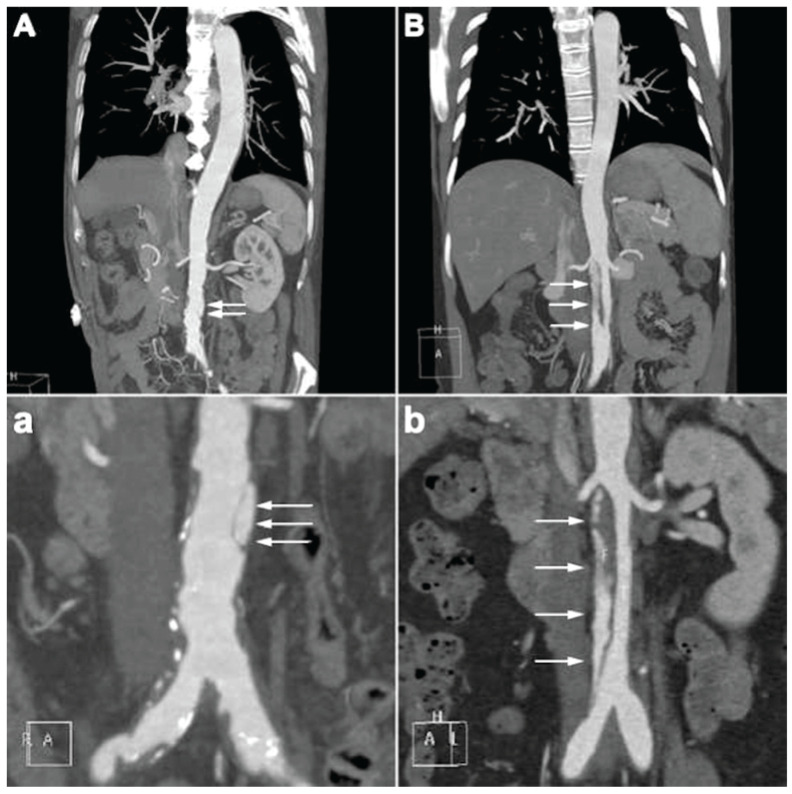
(A,a) the characteristic of focal-SIAAD on aortic CTA is the presence of a short intimal flap in the abdominal aorta, often with aortic arteriosclerosis. (B,b) the characteristic of extensive-SIAAD on aortic CTA is the presence of a long intimal flap in the abdominal aorta, normally without apparent aortic arteriosclerosis. Two partial enlarged images of (A,B) are shown in (a,b), respectively. White arrows denote the intimal flap and false lumen. CTA, computed tomography angiography; SIAAD, spontaneous isolated abdominal aortic dissection.
